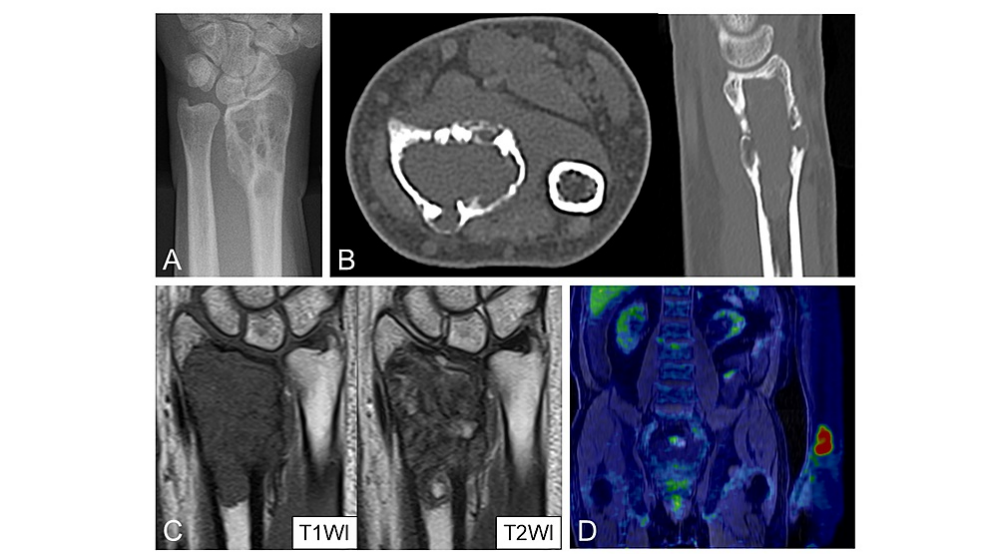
Images at the time of referral to our hospital. A: X-ray image showed a geographic radiolucent lesion with sclerotic margins. B: CT image depicted no calcification inside the tumor, and the bone cortex appeared to be partially disrupted. C: MRI images showed homogeneous isointense signals in the T1-weighted images and heterogeneously mixed iso- and high-signals in the T2-weighted images. D: FDG-PET image revealed high accumulation at the left distal radial metaphysis.
